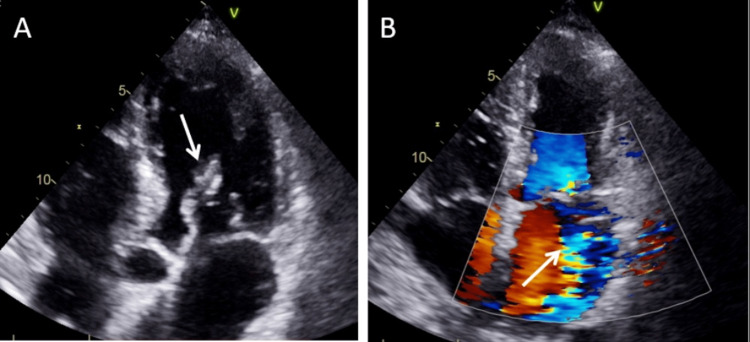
Comprehensive transthoracic echocardiogram Transthoracic echocardiogram (apical 5-chamber and 4-chamber views) performed by cardiac sonographer demonstrating (A) mass adherent to the anterior mitral valve leaflet (arrow) and (B) severe mitral valve regurgitation with color flow doppler (arrow).
